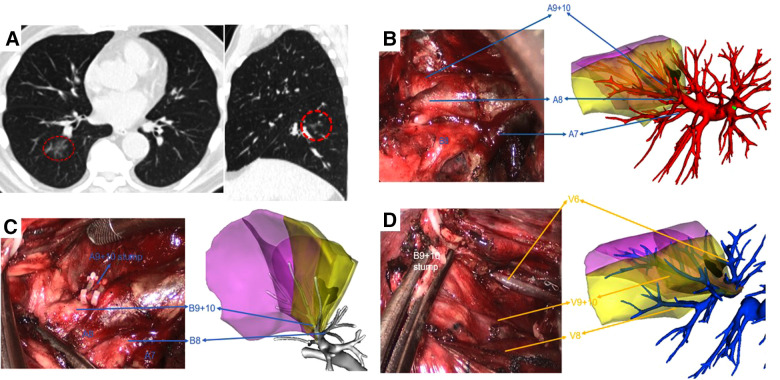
Right S9 + 10 VATS segmentectomy under real-time 3D reconstruction guidance. (A) preoperative CT scan showing a 10 mm GGN lesion in the right S9 + 10. (B) Branches of the pulmonary artery, (C) branches of the bronchus, and (D) branches of the pulmonary vein of the target segment were compared by using the real-time guidance of 3D reconstruction.
